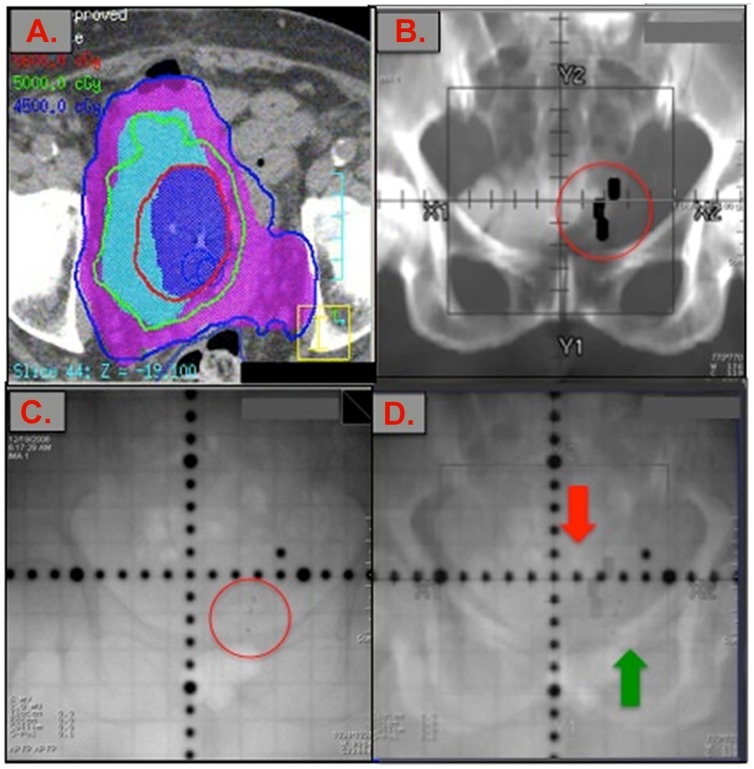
Computed Tomography (CT), Digitally Reconstructed Radiograph (DRR), and on-table Portal images with fiducial markers present. (A.) Computed Tomography (CT-scan) dosimetry imaging of pelvis with bladder fiducial markers in place. The gross tumor volume (GTV; dark blue) received 56-Gy in 25 fractions. The clinical tumor volume (CTV; light blue) equals the bladder and received 50 Gy in 25 fractions. The planning tumor volume (PTV; purple) equals the CTV+1 cm + the ipsilateral pelvic side-wall. (B.) Digitally reconstructed radiograph (DRR) of pelvis; shows the *anticipated* location of the three markers we placed at the tumor site (large black rectangles), based on the gold markers visible on planning CT. (C.) Gold markers seen on an AP portal image of pelvis, (*circled*), (D) Fusion of the DRR and AP portal images showing the predicted position for gold markers (red arrow) compared to the true position of the gold markers (green arrow) prior to any patient adjustment.
